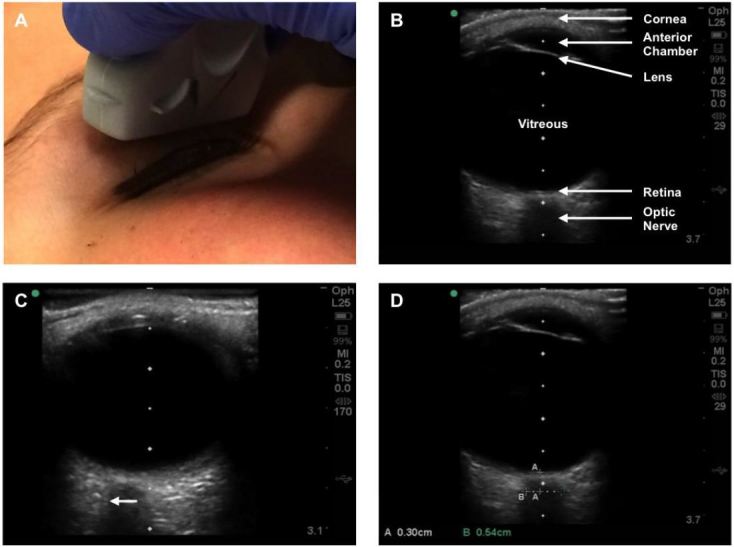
Ocular ultrasound was performed in the transverse plane using a linear transducer to identify the optic nerve (A and B). The nerve sheath was included in optic nerve sheath diameter (ONSD) measurements when visualized (C, arrow) and measurements were taken at a depth of 3 mm posterior to the globe (D).
